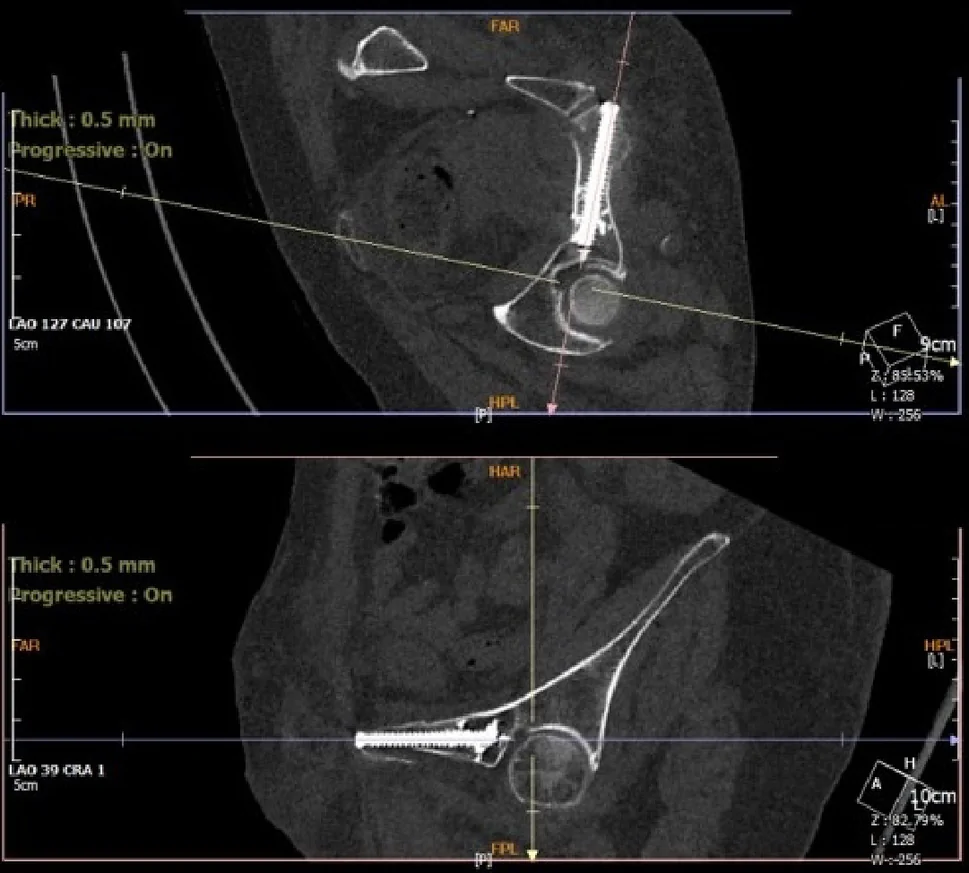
Multiplanar CT reconstruction Pubic ramus screw lt.* (Same patient as in figures 11 and 12). Source: Haida et al. (2025), *Die Unfallchirurgie* [16]. CC BY 4.0.
